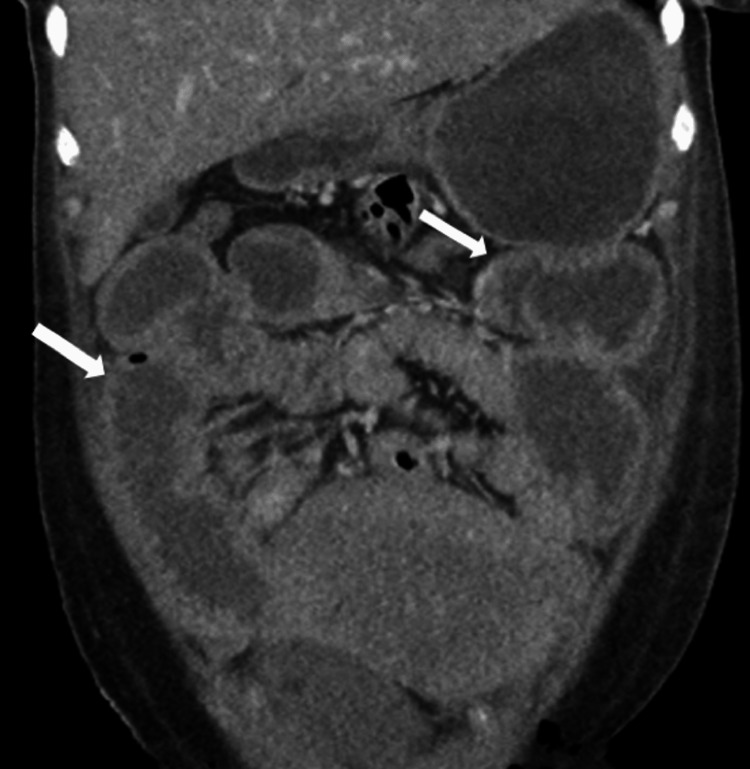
CT abdomen and pelvis also showed thickening of the small bowel and cecum, suggestive of a superimposed infectious or inflammatory enterocolitis
